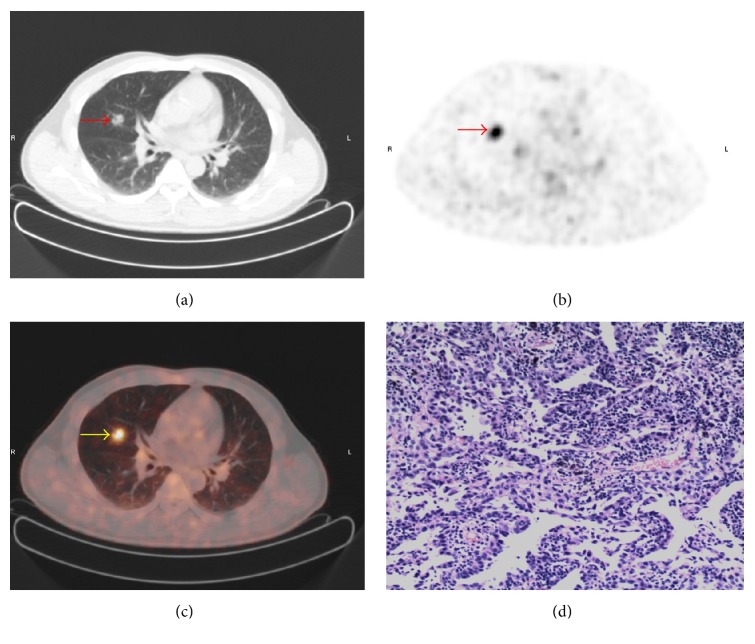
A typical PET/CT finding in a 54-year-old male patient. (a) On CT image, the nodule in the right middle lobe is observed (arrow); the maximum diameter of nodule is 16 mm. (b) On PET and (c) on PET/CT image, increased ^18^F-FDG uptake is detected; SUV_max_ = 3.9. (d) Pathologic examination suggests gland squamous cell carcinoma. The *y* value calculated by our model is 0.73. The result of our model is consistent with PET/CT and pathological results.
